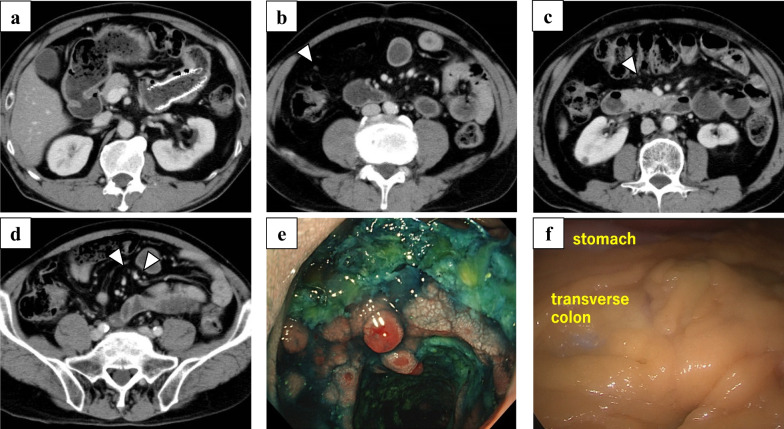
Six months after initiation of chemotherapy. a Computed tomography revealed the stent placed in the primary lesion, but shows no noticeable thickening of the surrounding walls. b–d Computed tomography showed extreme shrinkage of the patient’s peritoneal metastases (arrowhead). e Endoscopy found the growth of granulation in the stent lumen. Biopsy revealed no malignant findings. f The staging laparoscopy did not identify the metastatic nodules that were previously observed in the omentum
